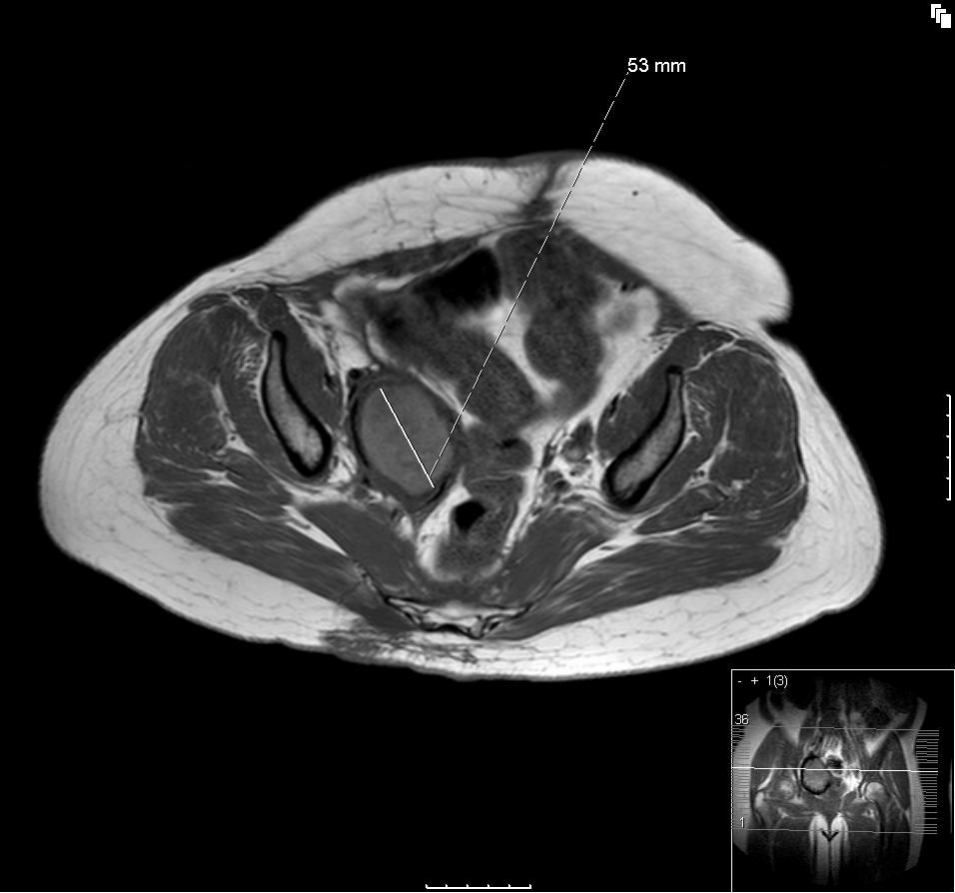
MRI scan of the pelvis after two years on sirolimus, demonstrating a reduction in size of the perivascular epithelioid cell tumor (PEComa) (measuring 53 mm) following treatment with sirolimus.
